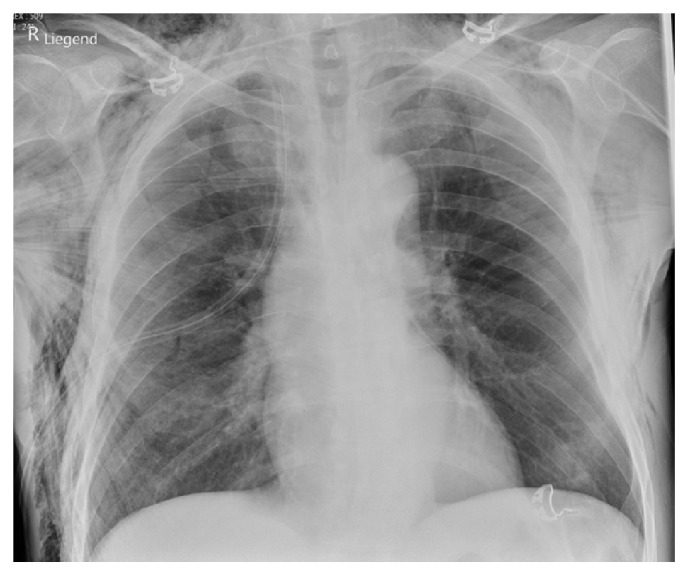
Chest X-ray after insertion of thorax drainage.
